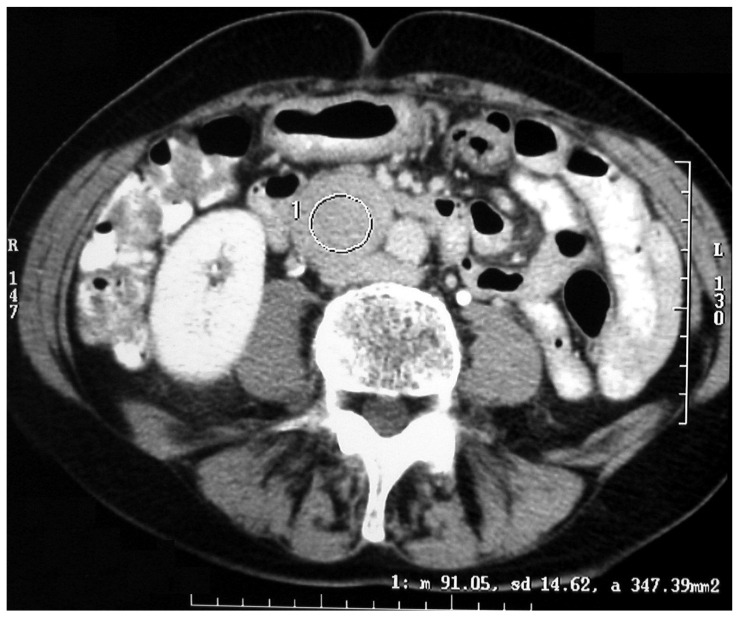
CT scan of an abdominal paraganglioma of above the aortic bifurcation (Zuckerkandl organ’s).
